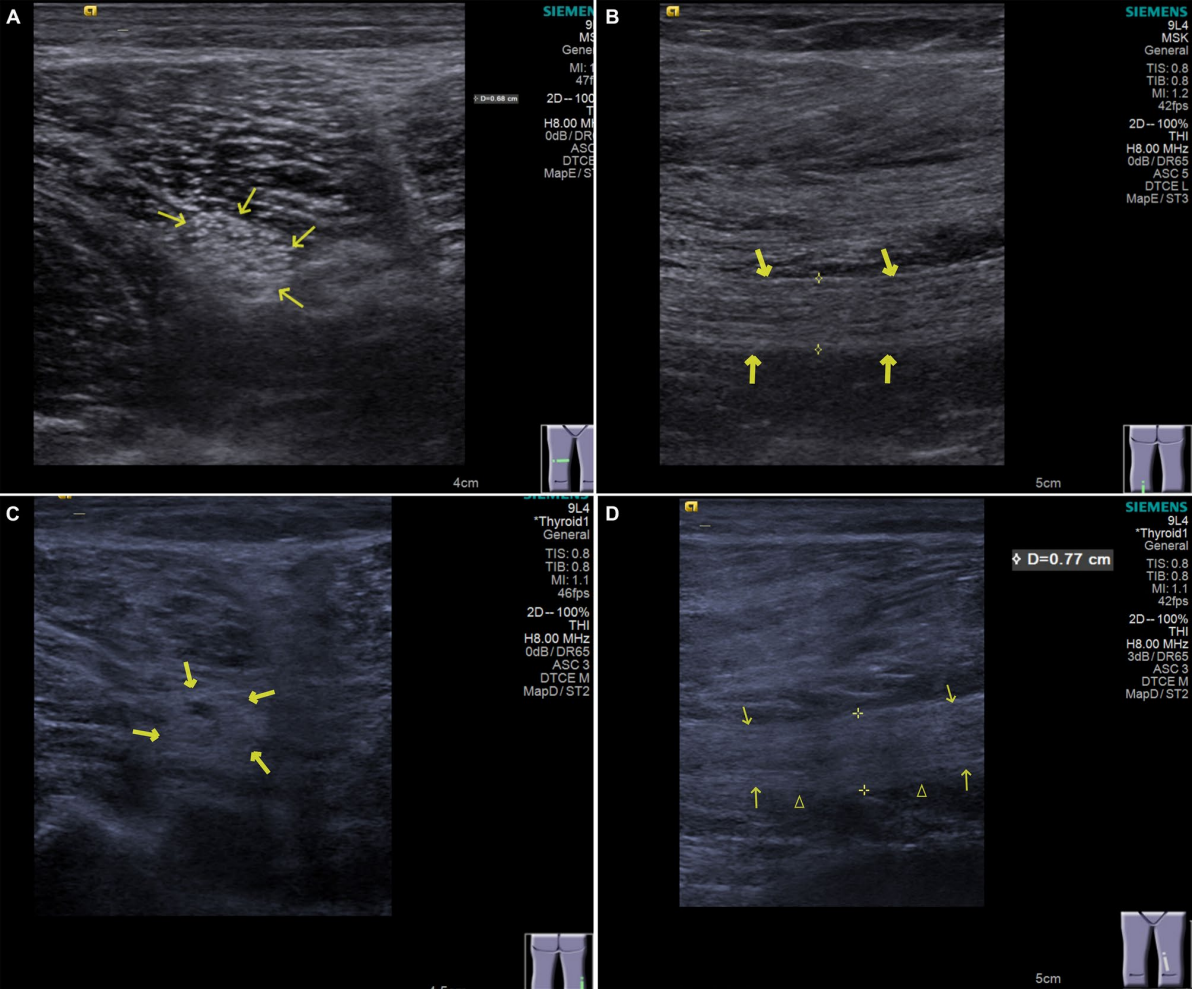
Grey-scale ultrasound images of DPN and T2DM patients. (A) The internal echoes of cross-sectional B-ultrasound images of T2DM show a regular honeycomb pattern (presented by arrows). (B) The longitudinal section image of T2DM patients (presented by arrows). (C) The internal echoes in the cross-sectional images of DPN patients show liquefaction (presented by arrows). (D) The longitudinal section image of DPN patients. The nerve bundle between the triangles is enlarged and blurred texture, arrows indicate the width and clear texture of normal nerve bundle
